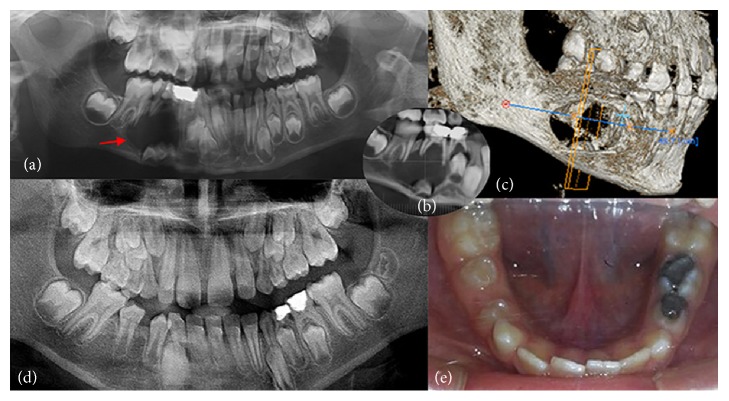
Case 4. (a)–(c) Initial panoramic X-ray image (a) and CBCT images (b)-(c) indicating a large DC in the right maxillary region. (d)-(e) 26-month follow-up X-ray image and intraoral photo showing the complete healing of the cystic region and eruption of the affected tooth.
